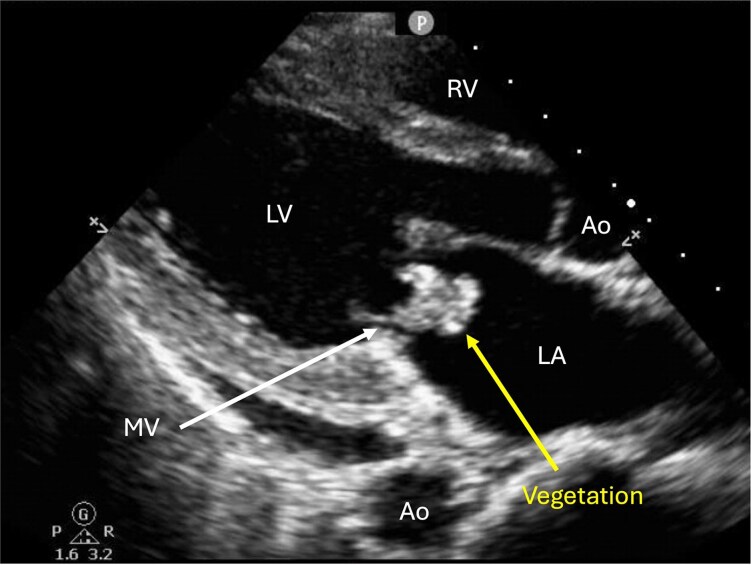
Case 1 (Mitral Valve Vegetation): transthoracic echocardiogram (TTE) in parasternal long axis. The lobulated, irregular, mobile mass seen attached to the atrial side of the mitral valve represents a vegetation, consistent with the diagnosis of infective endocarditis. This figure corresponds to Supplementary material online, *Video S1*. Ao, aorta; LA, left atrium; LV, left ventricle; MV, mitral valve; RV, right ventricle.
